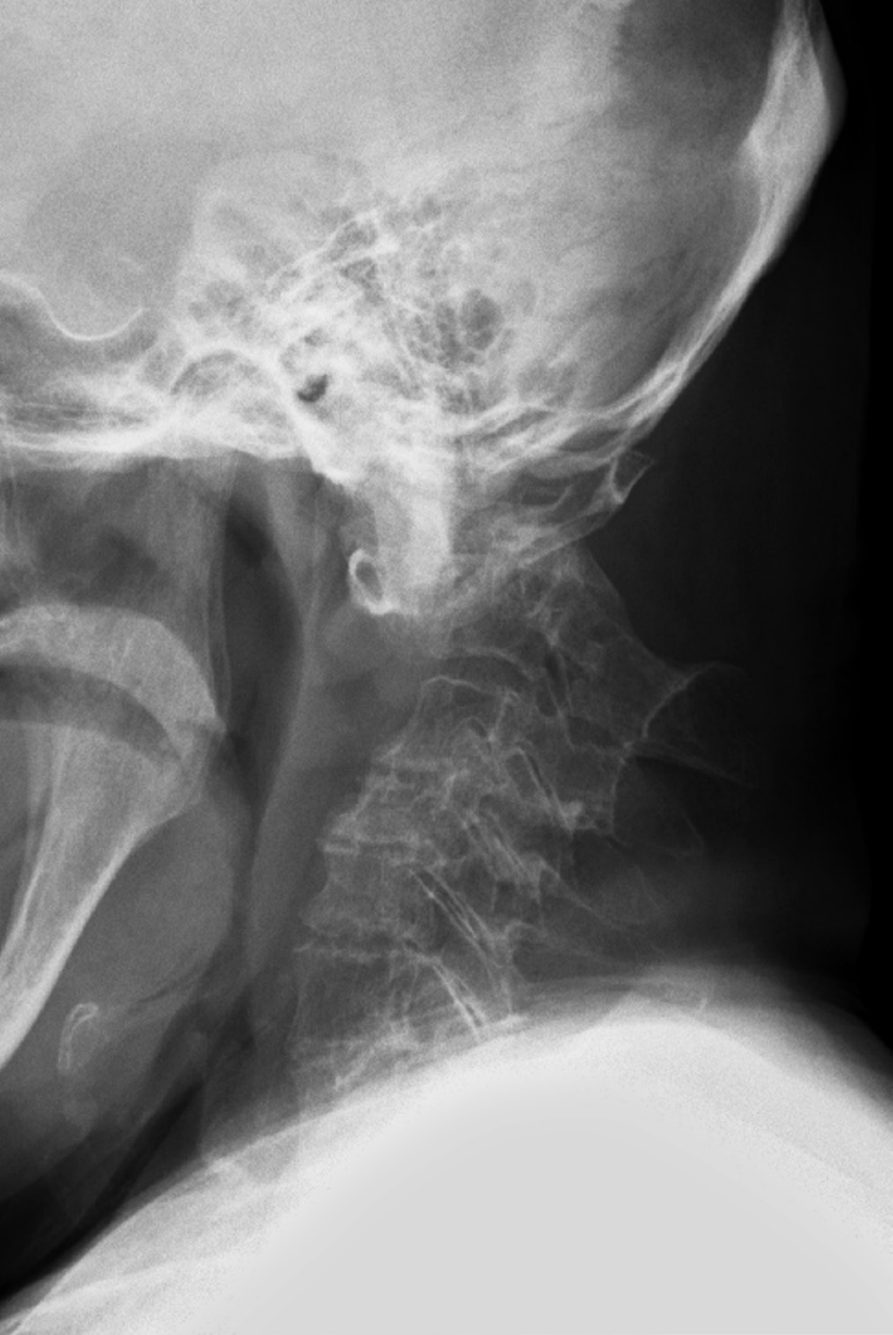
A plain radiograph of the lateral cervical spine taken at our hospital revealed an atlanto-axial (C1–2) dislocation secondary to an odontoid fracture.
